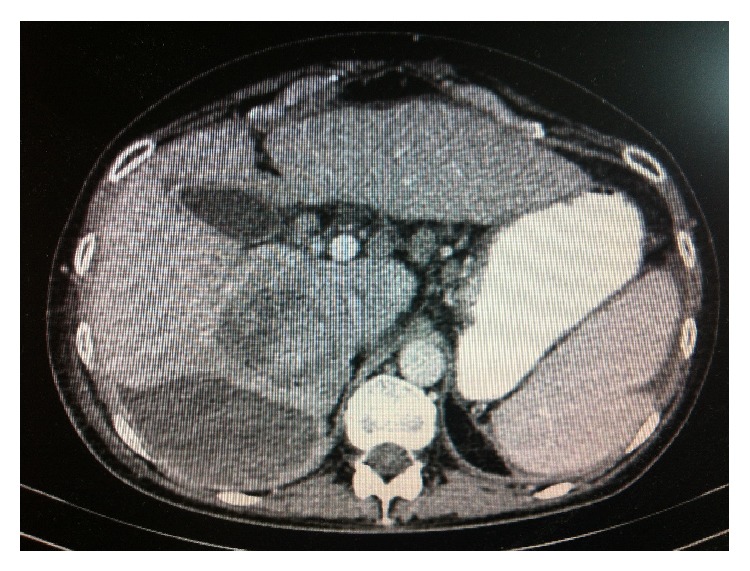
A three-phase CT of the abdomen. In a 4-segment focal lesion with typical enhancement in contrast phase and washout effect in a venous phase.
